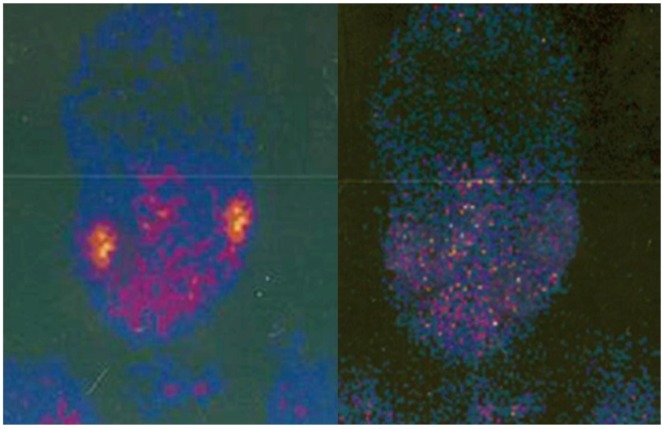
Left, pre-operative sialoscintigraphy; Right, sialography 15 days after btx injections.
